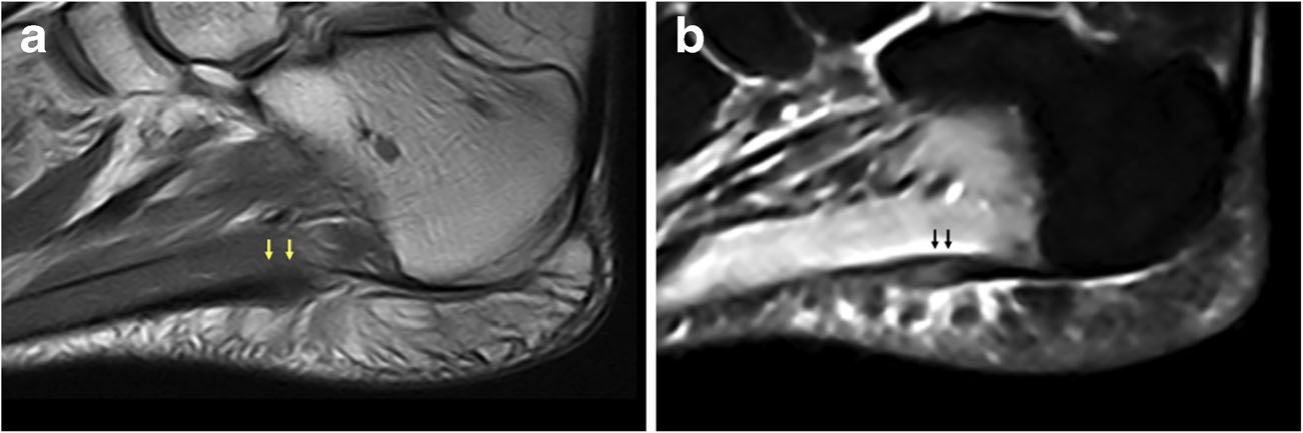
Plantar xanthoma. On both sagittal T1-weighted (a) and fluid-sensitive (b) images, xanthoma (*arrows*) appears as fusiform enlargement of the PF and shows heterogeneous signal intensity
